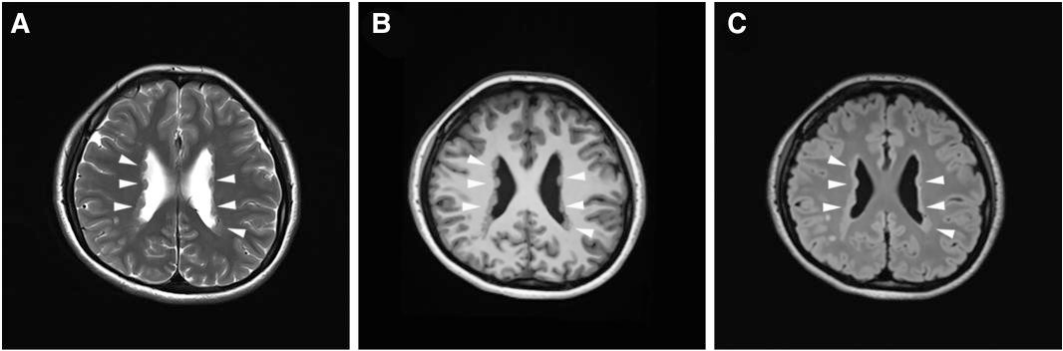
Imaging features of periventricular nodular heterotopia (PVNH). PVNH patient: MRI T2-weighted (A), multiplanar reformation (MPR; B) and T2-weighted dark fluid (C) images reveal abnormal nodules of grey matter (PVNH) adjacent to the bilateral lateral ventricles (indicated by arrows).
